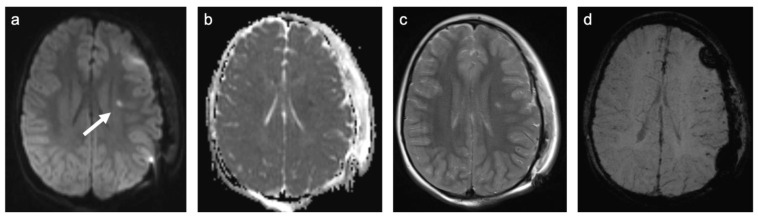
Representative case “Traumatic white matter injuries”: diffuse axonal injury. DWI/ADC (a,b), T2W (c), and Susceptibility weighted imaging (SWI; (d)) sequences. Fourteen-year-old girl admitted after a traumatic car accident during which she was ejected from her seat. MRI shows a diffusion restricted, diffuse axonal injury lesion in the lobar white matter ((a–c), white arrow). SWI demonstrates small regions of susceptibility artefacts at the grey–white matter junction corresponding to cerebral microbleeds (d). Additional findings are a subgaleal fluid collection (left side) following craniotomy for treatment of cranial impression fracture.
